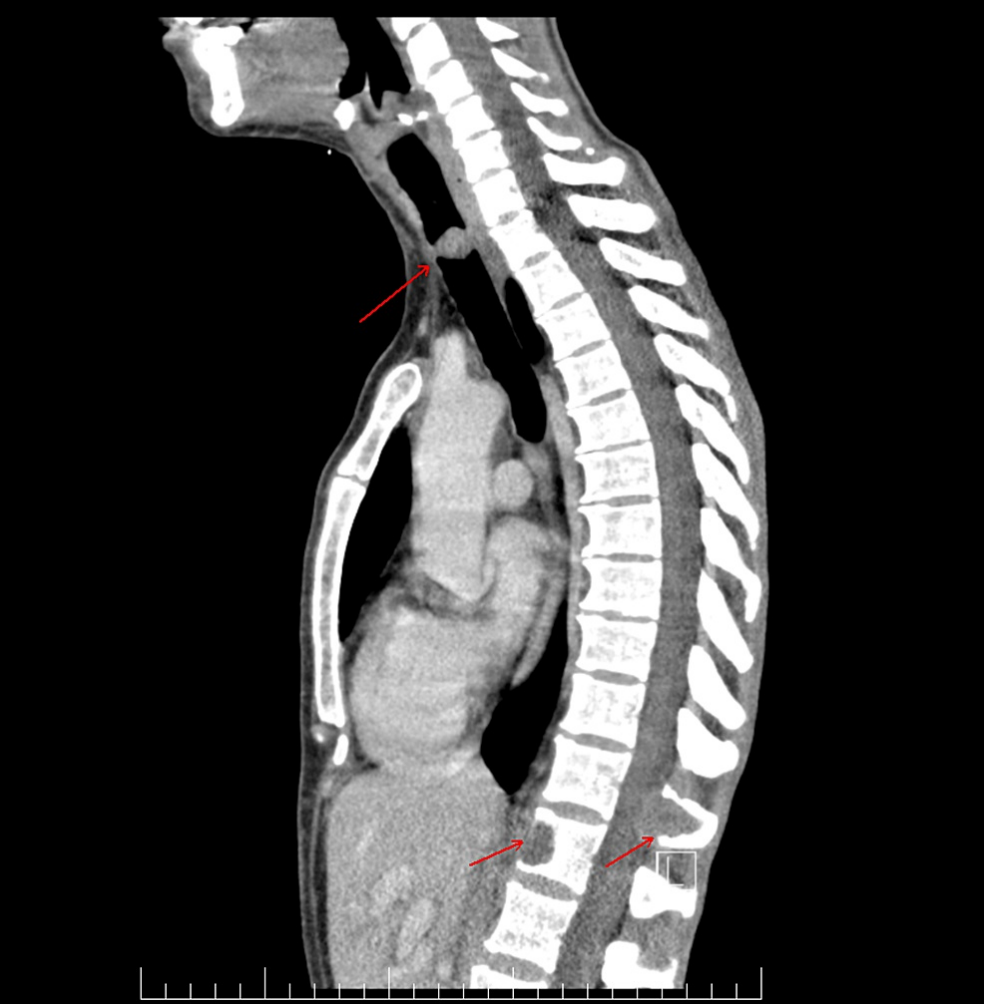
Sagittal view of the spinal cord CT. A vegetative round intraluminal tracheal lesion measuring 15 mm in the anteroposterior diameter, 15 mm in laterolateral diameter, and 13 mm in craniocaudal diameter, creating a quasi-complete obstruction of the tracheal lumen. This lesion is situated at the level of the cervical trachea, specifically at the C7-T1 vertebrae. An osteolytic lesion is observed at the level of the posterior arch of the T11 vertebral body, measuring 30 mm in anteroposterior diameter, 26 mm in laterolateral diameter, and 29 mm in craniocaudal diameter. It invades the posterior epidural space, demonstrates neuroforaminal invasion, and results in vertebral canal stenosis at this level (anteroposterior diameter = 70 mm, compared to 13 mm above and below). Another osteolytic lesion is described in the 1/3 anterior part of the T12 vertebral body, measuring 13 mm in anteroposterior diameter and 21 mm in craniocaudal diameter.
